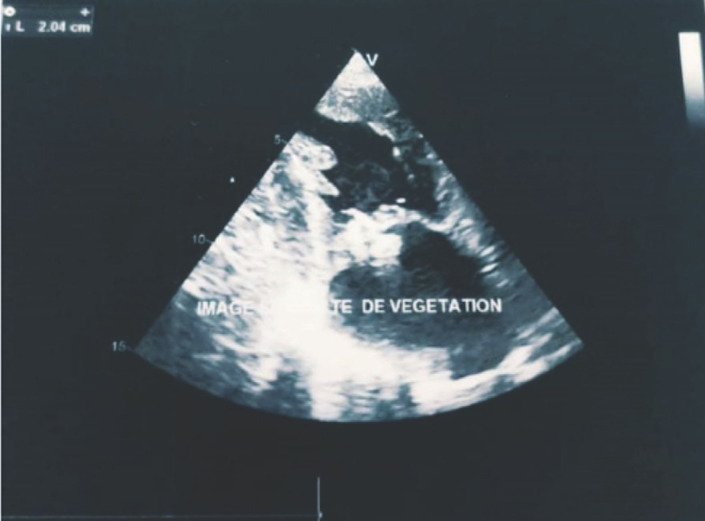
Grosse végétation de 20 mm sur la grande valve mitrale à l’échocardiographie 20 mm thick vegetation on the large mitral valve on echocardiography
